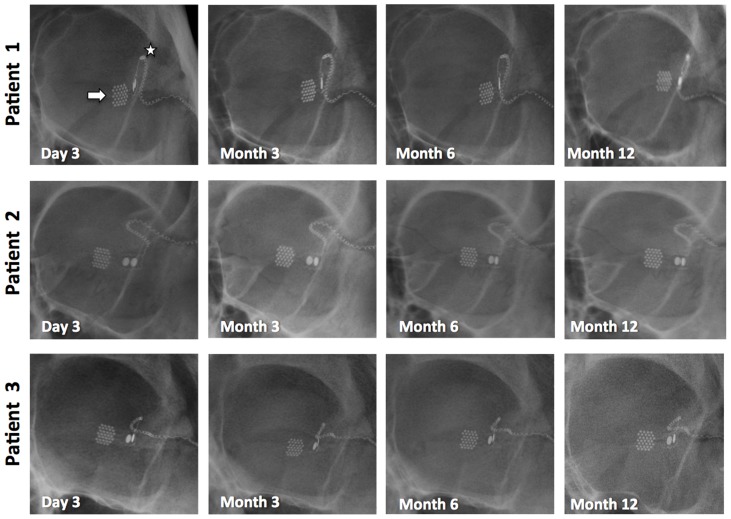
Stability of the intraocular array (arrow) and helical lead wire (star) over the initial 12 months of implantation, as documented by X-ray images. Additional scans were taken to monitor the percutaneous connector (not shown), which also stayed stable over this time period.
